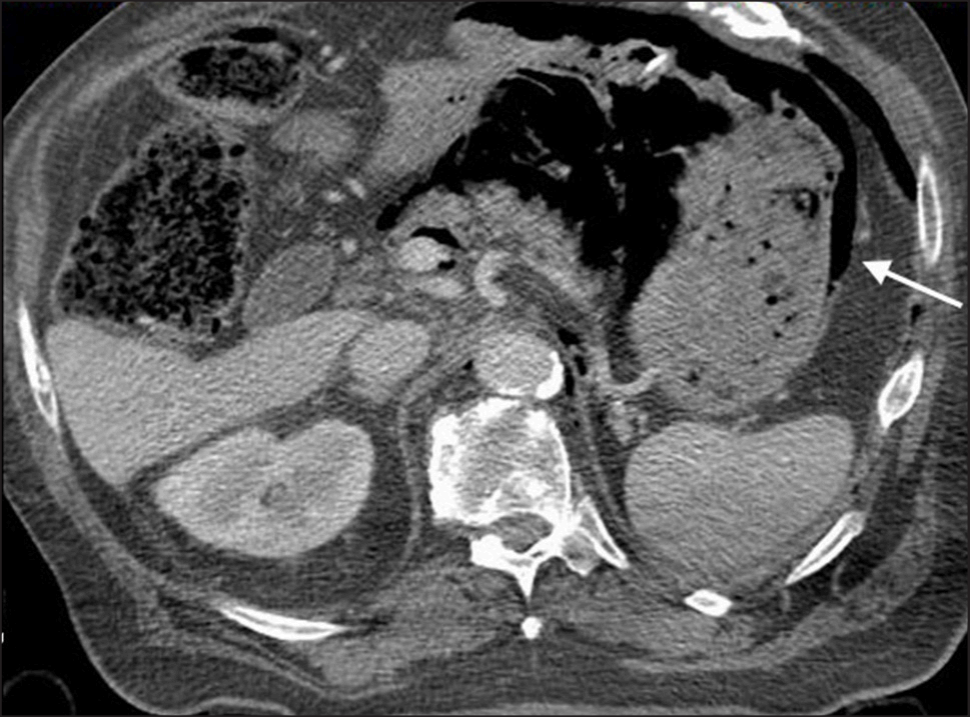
Contrast-enhanced CT, axial slice. Evidence of gastric intramural gas (arrow) and air bubbles within the retroperitoneal space.
